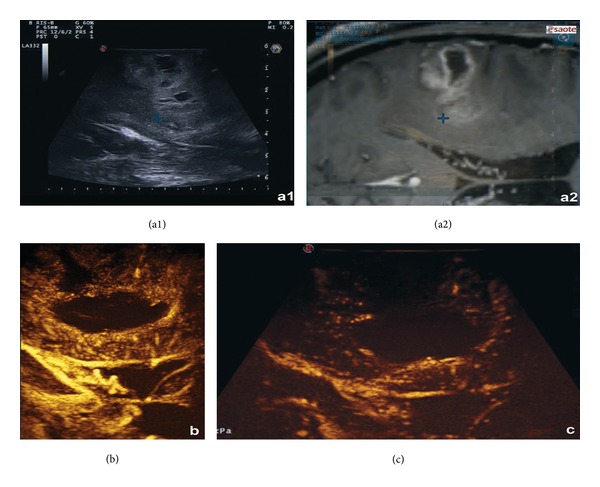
Intraoperative control of a right frontal GBM, using fusion imaging between intraoperative US (a1) and preoperative MRI (a2) linked via a navigated US probe with a virtual navigation system: (a1) shows a hyperechoic superficial lesion, with ill-defined borders and microcystic areas and in (a2) the corresponding MRI imaging is displayed. In panel (b) the B-mode imaging is enhanced with a contrast agent, showing a superficial nodular enhancement with a deeper ring enhancement delimitating a nonperfused necrotic central area. Medullary draining veins are also visible, draining towards the ependymal zone. In panel (c) the postresection control with CEUS shows the absence of the nodular ring enhancement, without contrast enhancement along the wall of the surgical cavity.
